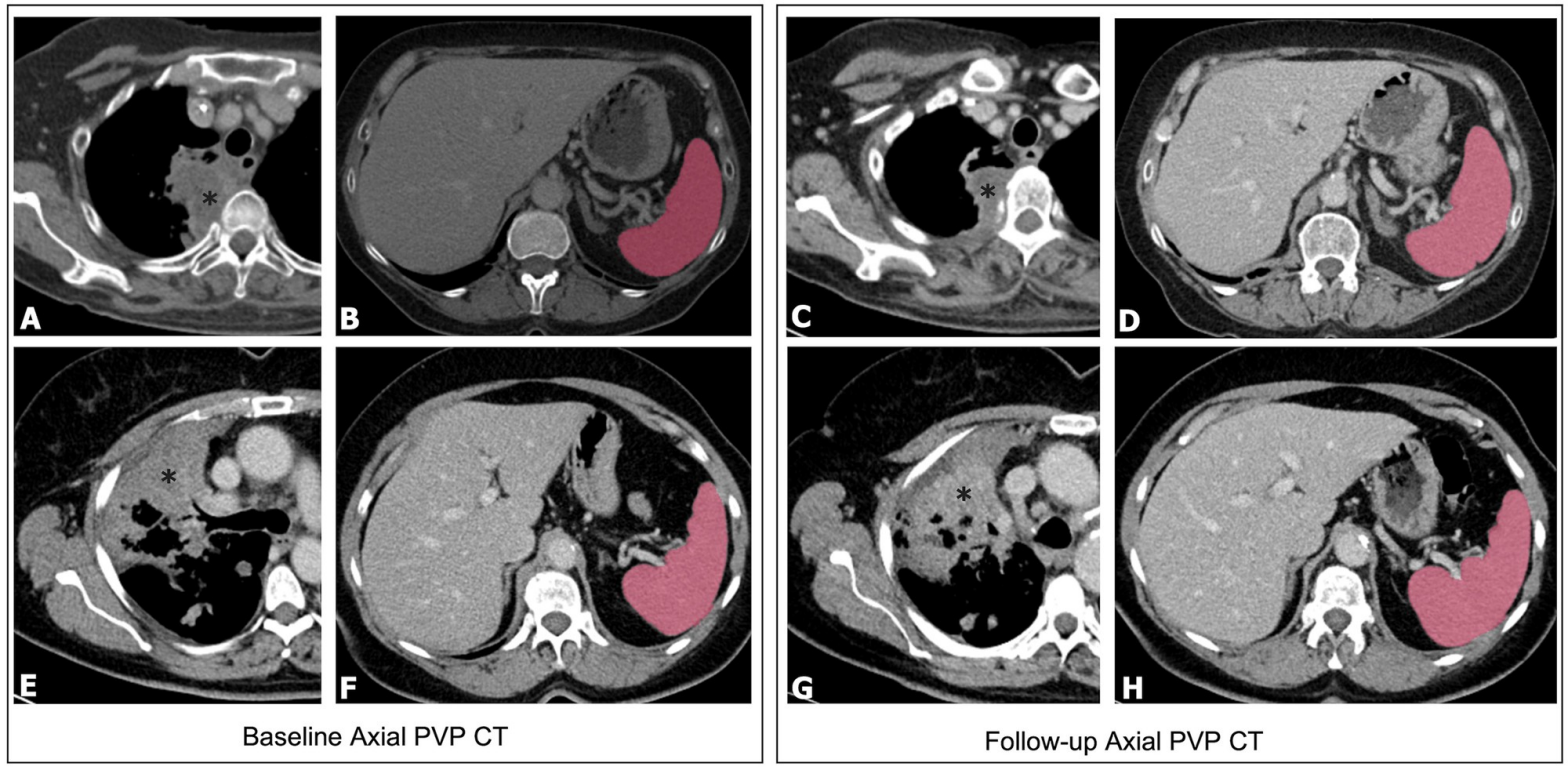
Representative computed tomography (CT) images used to calculate spleen volume. Non-small cell lung cancer (*). Baseline axial CT scans and follow-up scans in a responder patient (A-D) and a non-responder (E-H).
